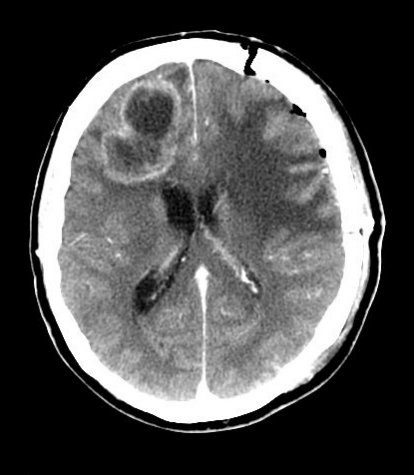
Brain Computed Tomography performed 24 h after first surgical intervention.
